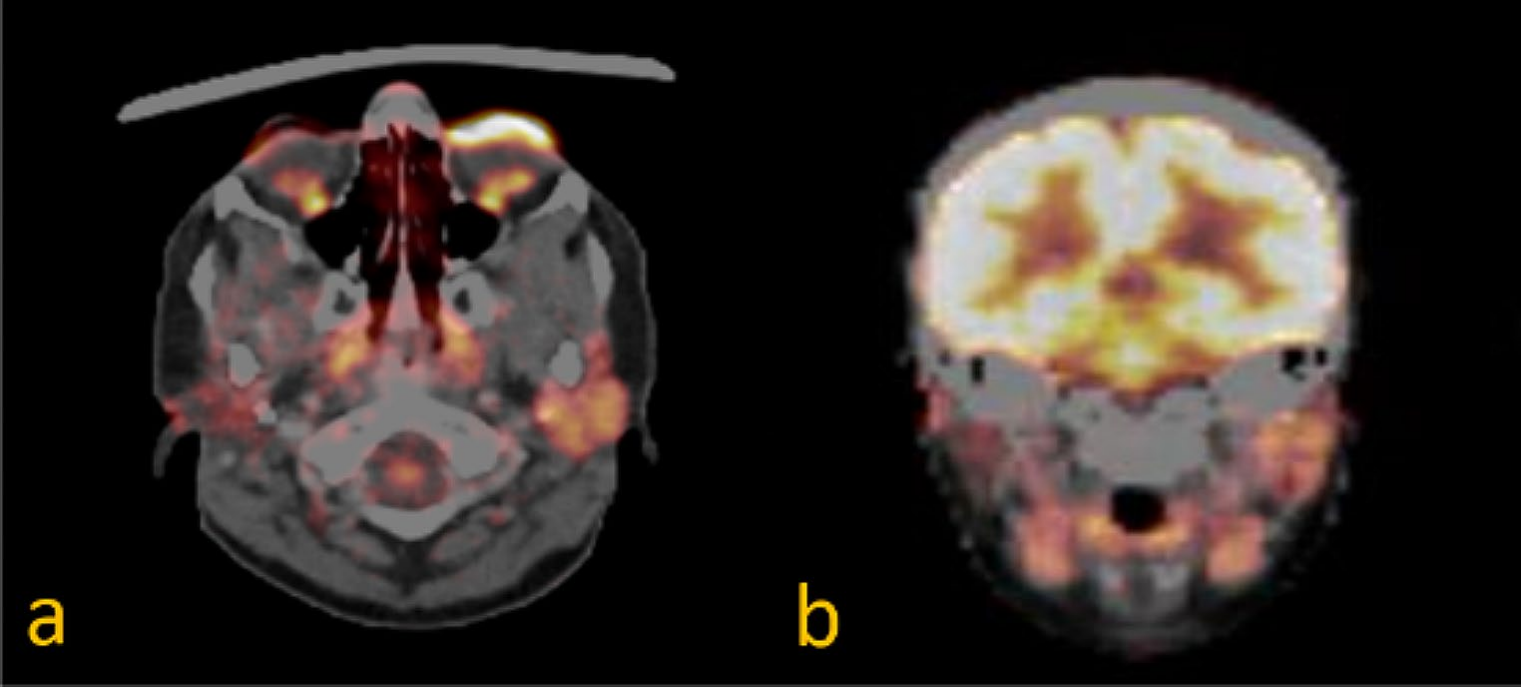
(a) 18-FDG PET-CT transversal; (b) 18-FDG PET-CT coronal; the right parotid gland shows even lower 18-FDG tracer uptake than the left side
